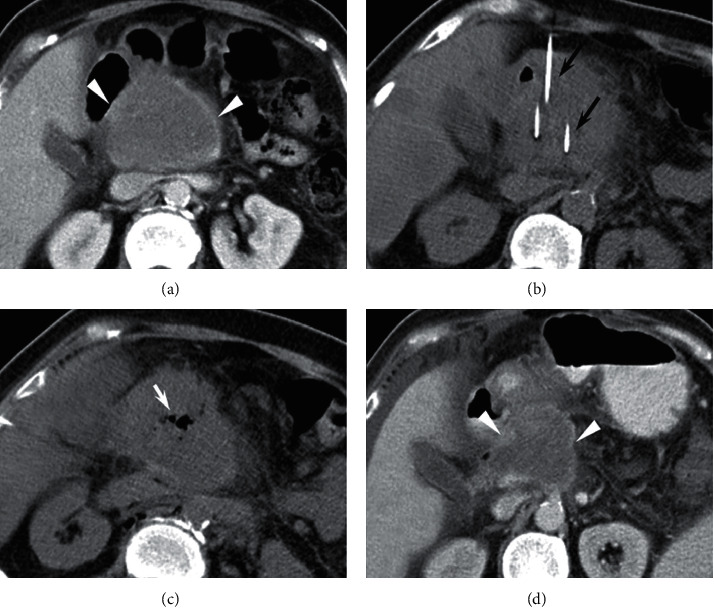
A 66-year-old female with unresectable pancreatic head tumors treated with gemcitabine and irreversible electroporation (GEM-IRE) simultaneously. (a) Preoperative contrast-enhanced CT showed tumor size (7.4 × 6.2 cm). (b) Arrows indicate electrode probes. (c) Bubbles were seen immediately after IRE. (d) 3 months after operation, preoperative contrast-enhanced CT revealed a tumor size of 4.6 × 5.1 cm.
